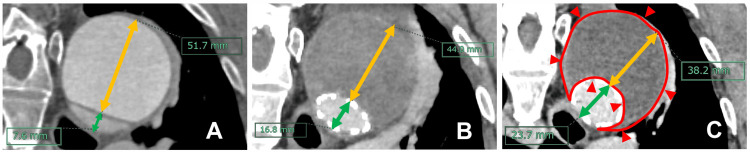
Serial contrast-enhanced computed tomography (CT) images of case 2. A: Preoperative CT. The true lumen diameter was 7.6 mm (green line), while that of the false lumen was 51.7 mm (yellow line). B: CT before the patient’s discharge from the hospital. The true lumen had expanded to 16.8 mm (green line), while that of the false lumen had decreased to 44.0 mm (yellow line). Favorable false lumen thrombosis was observed, accompanied by false lumen shrinkage and true lumen expansion. C: CT performed at six months postoperatively. The true lumen diameter had further increased to 23.7 mm (green line), while that of the false lumen had shrunk to 38.2 mm (yellow line). The false lumen remained well-thrombosed (the red outlined area), with continued false lumen shrinkage and true lumen enlargement.
